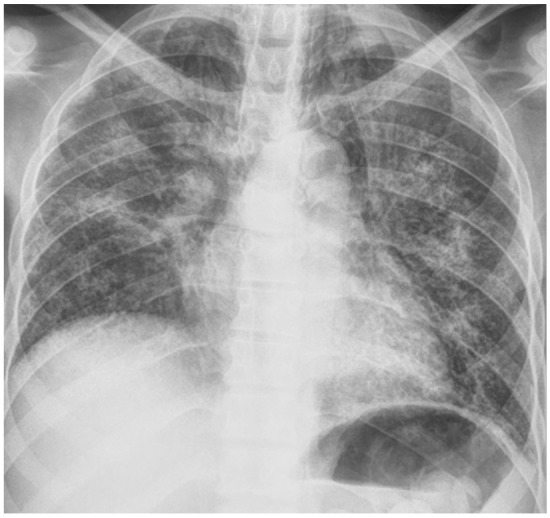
Follow-up chest radiograph – coarse bilateral patchy lung opacities with progression of reticular opacities
